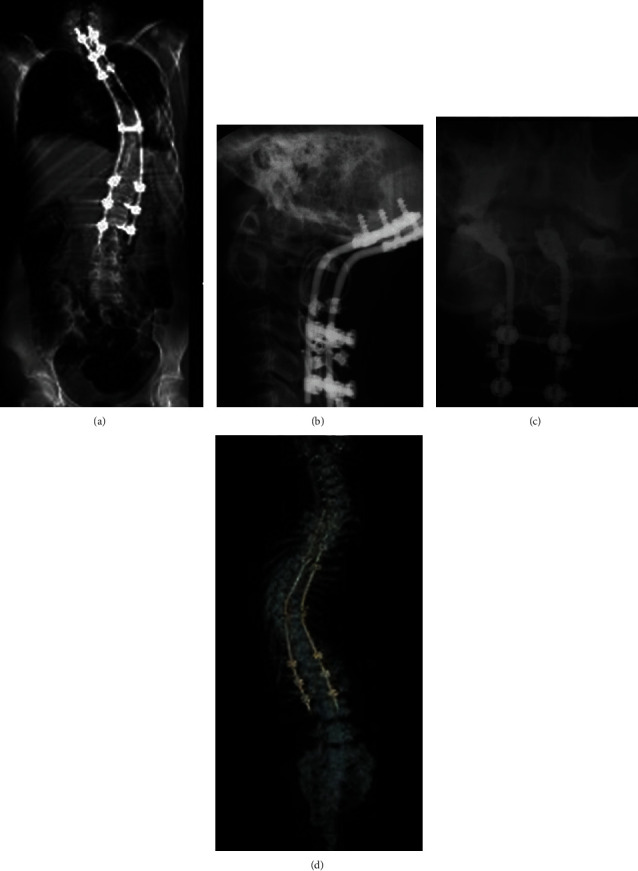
(a–c) Examples of X-rays showing a proper alignment of the spine at the cervical, thoracic, and lumbar levels with stabilization hardware consisting of bilateral rods and transpedicular screws. (d) 3D reconstruction of CT images depicting the hardware located in a case of scoliosis.
